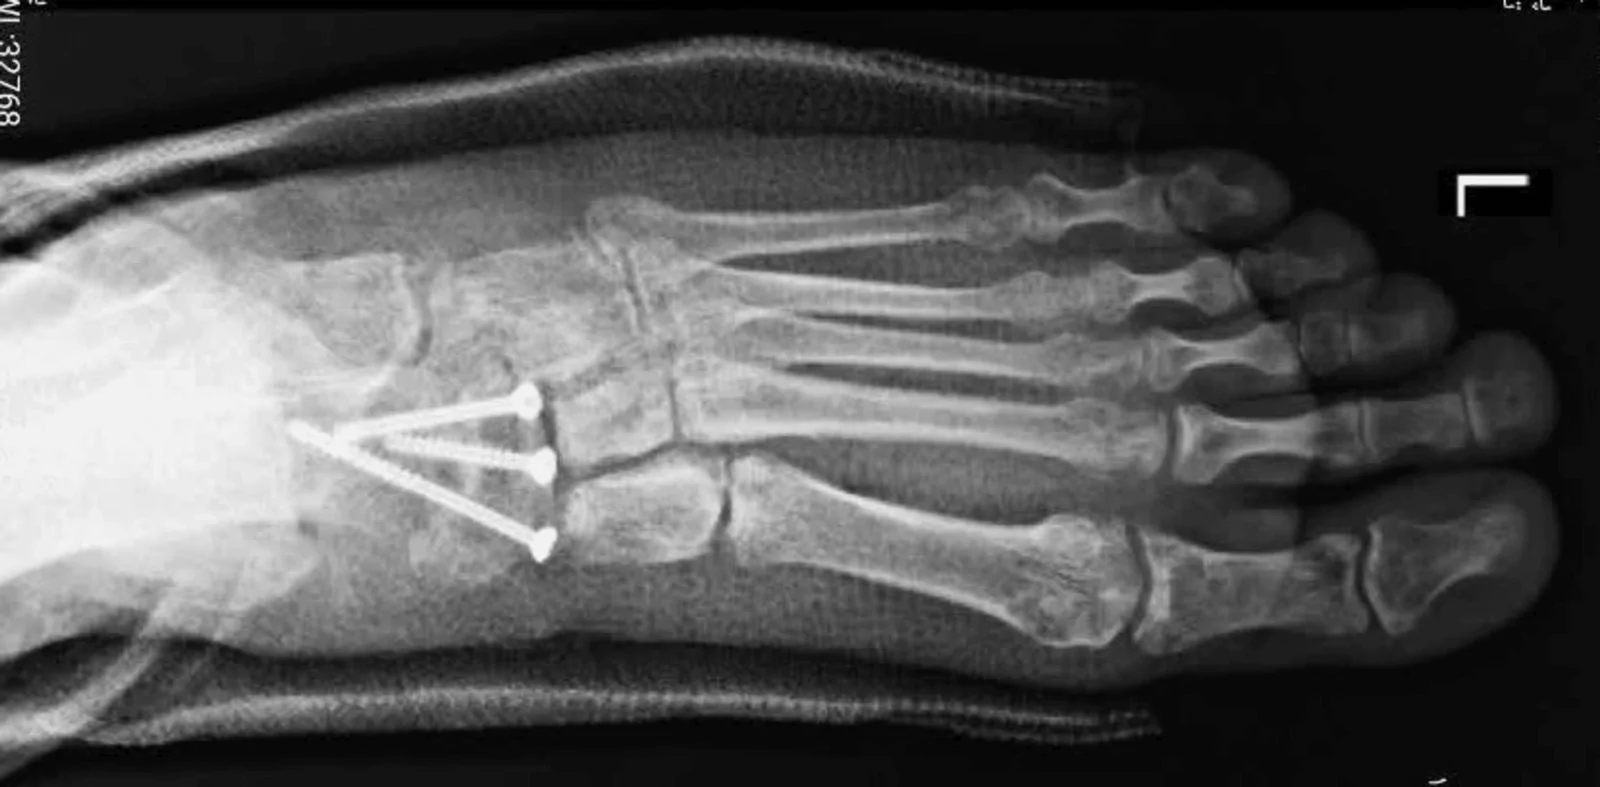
Postoperative radiograph one month after surgery
